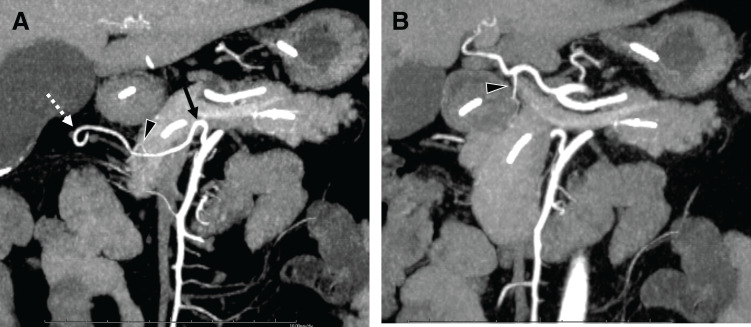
Preoperative multidetector CT. (A) Coronal CT image of the abdomen shows the GDA (arrow) originating directly from the SMA. The GDA gives rise to the RGEA (dotted arrow) and ASPDA (arrowhead). (B) The PSPDA (arrowhead) arising from the CHA, is visible as a very thin vessel. ASPDA, anterior superior pancreaticoduodenal artery; CHA, common hepatic artery; GDA, gastroduodenal artery; PSPDA, posterior superior pancreaticoduodenal artery; RGEA, right gastroepiploic artery; SMA, superior mesenteric artery
